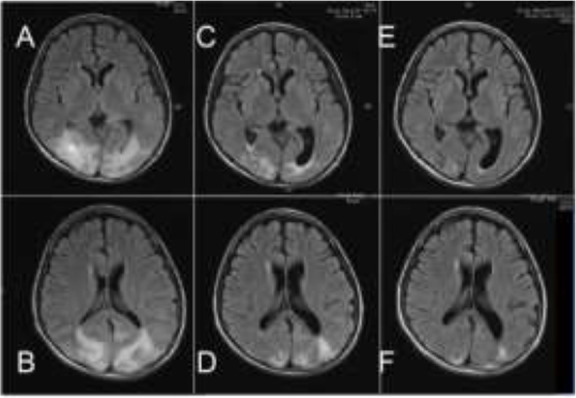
Serial MRI studies on day 61(A/B), 75(C/D) and 113(E/F)
